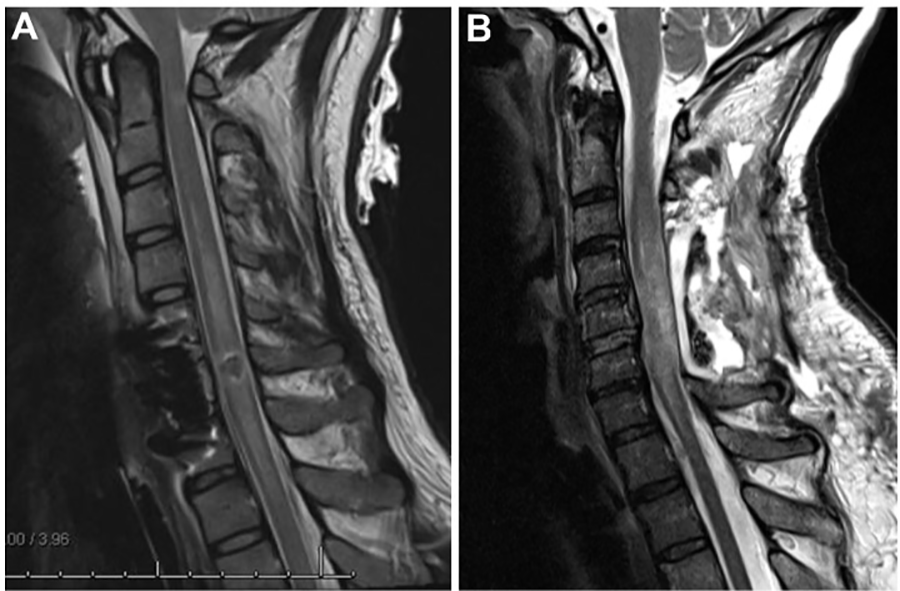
Examples of post-operative MRI to assess patency of SAS after decompression surgery. (A) Here, an anterior cervical vertebrectomy of C5 was performed, and the post-operative MRI shows that the SAS is patent around the injury site. In this individual, the ITP waveform that was either dampened pulsatile or pulsatile in 97% of the ITP waveform observations, and a total of 1,998 cc of CSF drained. (B) Here, a laminectomy of C3, C4, C5, and C6 was performed, but the post-operative MRI suggests that the SAS is occluded (in this case, the occlusion appears to be caused not only by spinal cord swelling but also posterior epidural fluid/blood). In this individual, the ITP waveform was flat in 97% of the ITP waveform observations, and only 134.5 cc of CSF were drained in the entire monitoring period. *Abbreviations:* CSF, cerebrospinal fluid; ITP, intrathecal pressure; MRI, magnetic resonance imaging; SAS, subarachnoid space.
